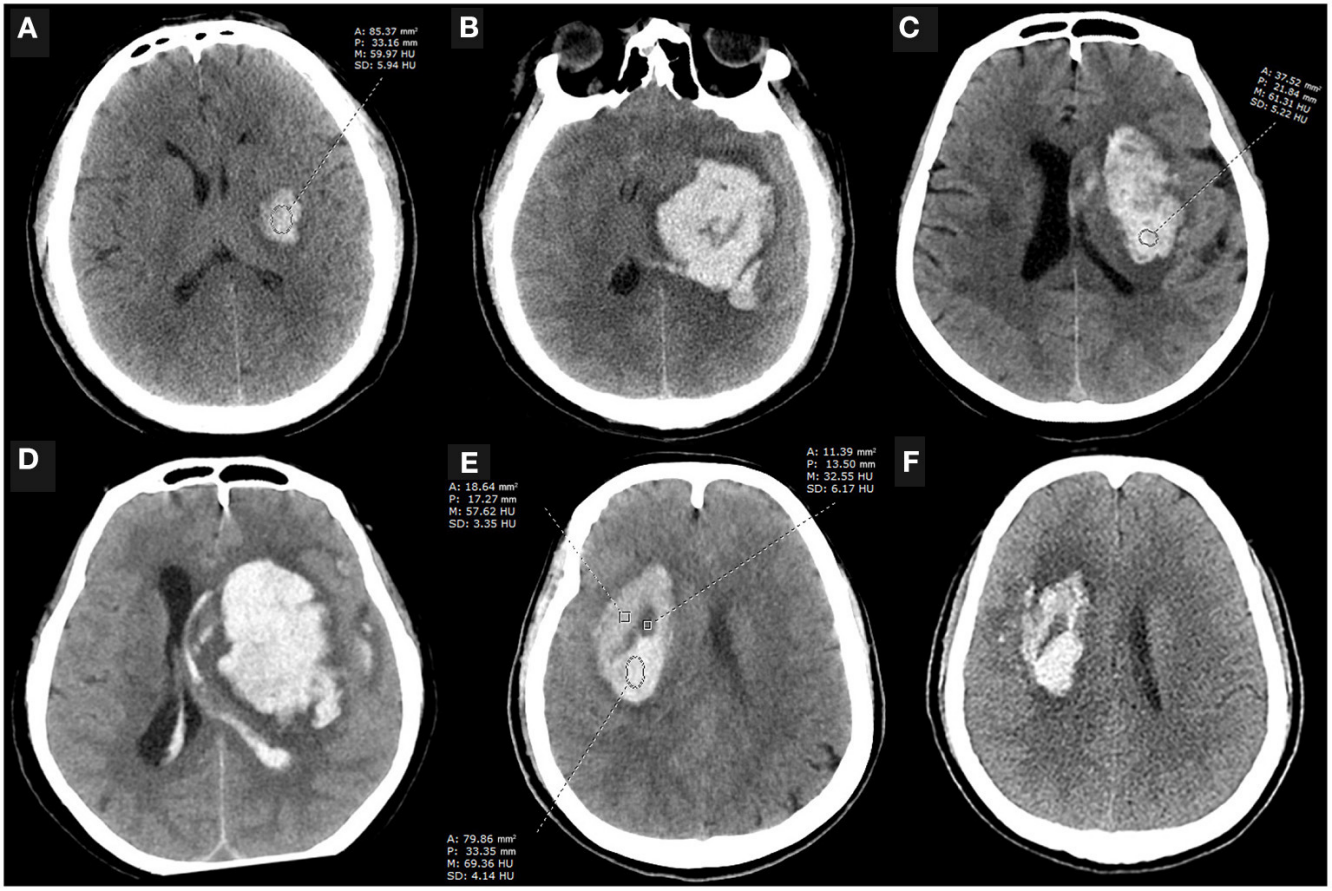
Case examples. (A,B) An initial NCCT scan performed 3.5 h after the onset of symptoms showed a small, regular shape hematoma with an attenuation value of 61.72 HU at the internal capsule, which enlarged 10 h later. The patient eventually died. (C,D) An NCCT scan performed 0.5 h after symptom onset showed a basal ganglia hematoma that was heterogeneous with an attenuation value of 63.86 HU within the non-hypodense region and appeared significantly expansion 12 h later. (E,F) NCCT scan performed 1 h after onset showed a heterogeneous basal ganglia hematoma with an attenuation value of more than 64 HU in the non-hypodense region, and the patient did not present with HE despite the presence of the black hole sign on the initial NCCT examination. A, area; P, perimeter; M, mean; SD, standard deviance; HU, Hounsfield units; NCCT, non-contrast computed tomography.
